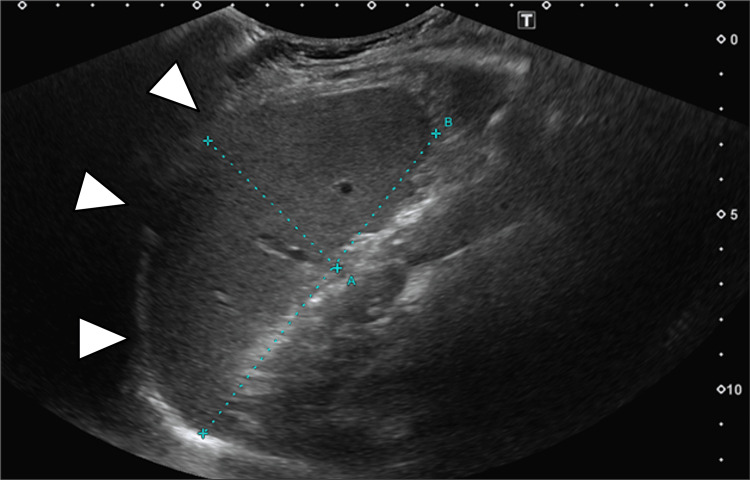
Abdominal Ultrasonography at Initial Presentation Abdominal ultrasonography at the initial presentation revealed mild splenomegaly, with the spleen measuring 109×52 mm (arrow heads). In Japanese adults, the normal splenic length on ultrasonography is generally ≤100 mm.
